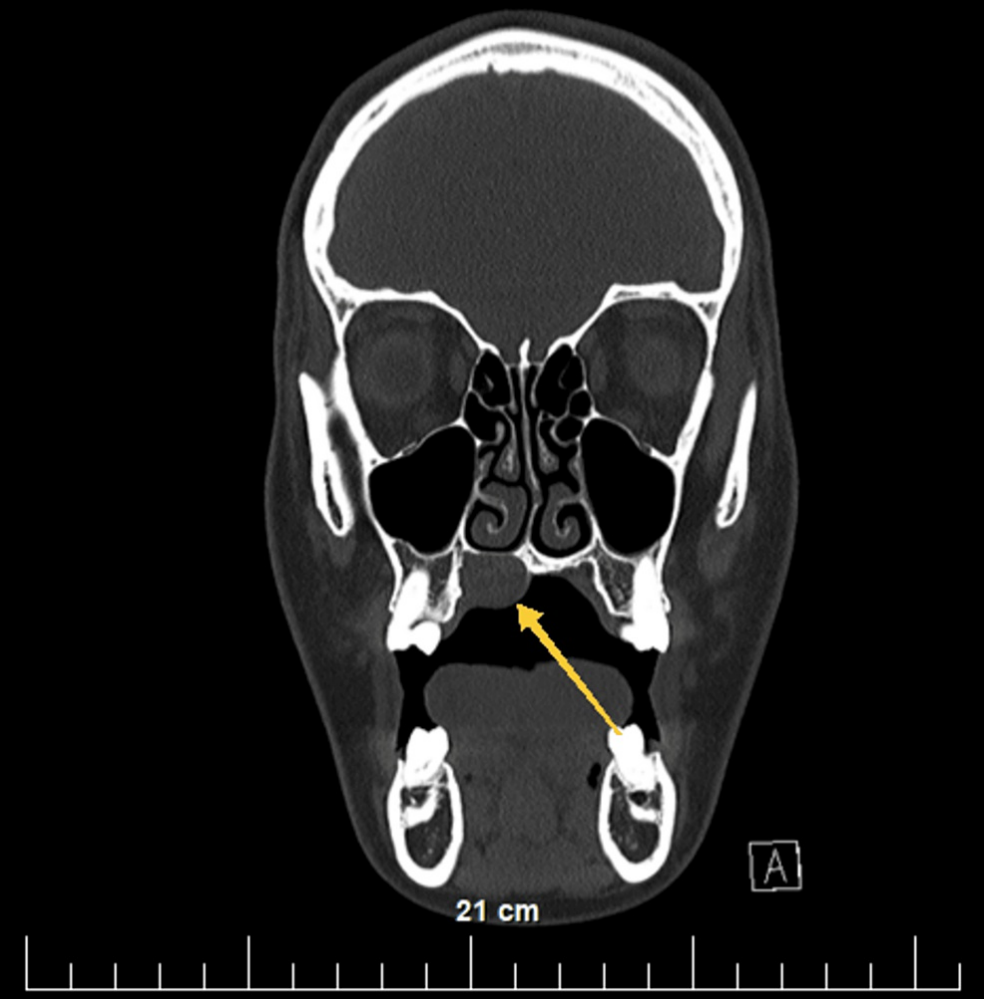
Transverse CT section of the head after intravenous administration of contrast material. A yellow arrow shows the location of the lesion on the right hard palate and the bony plate thinning
